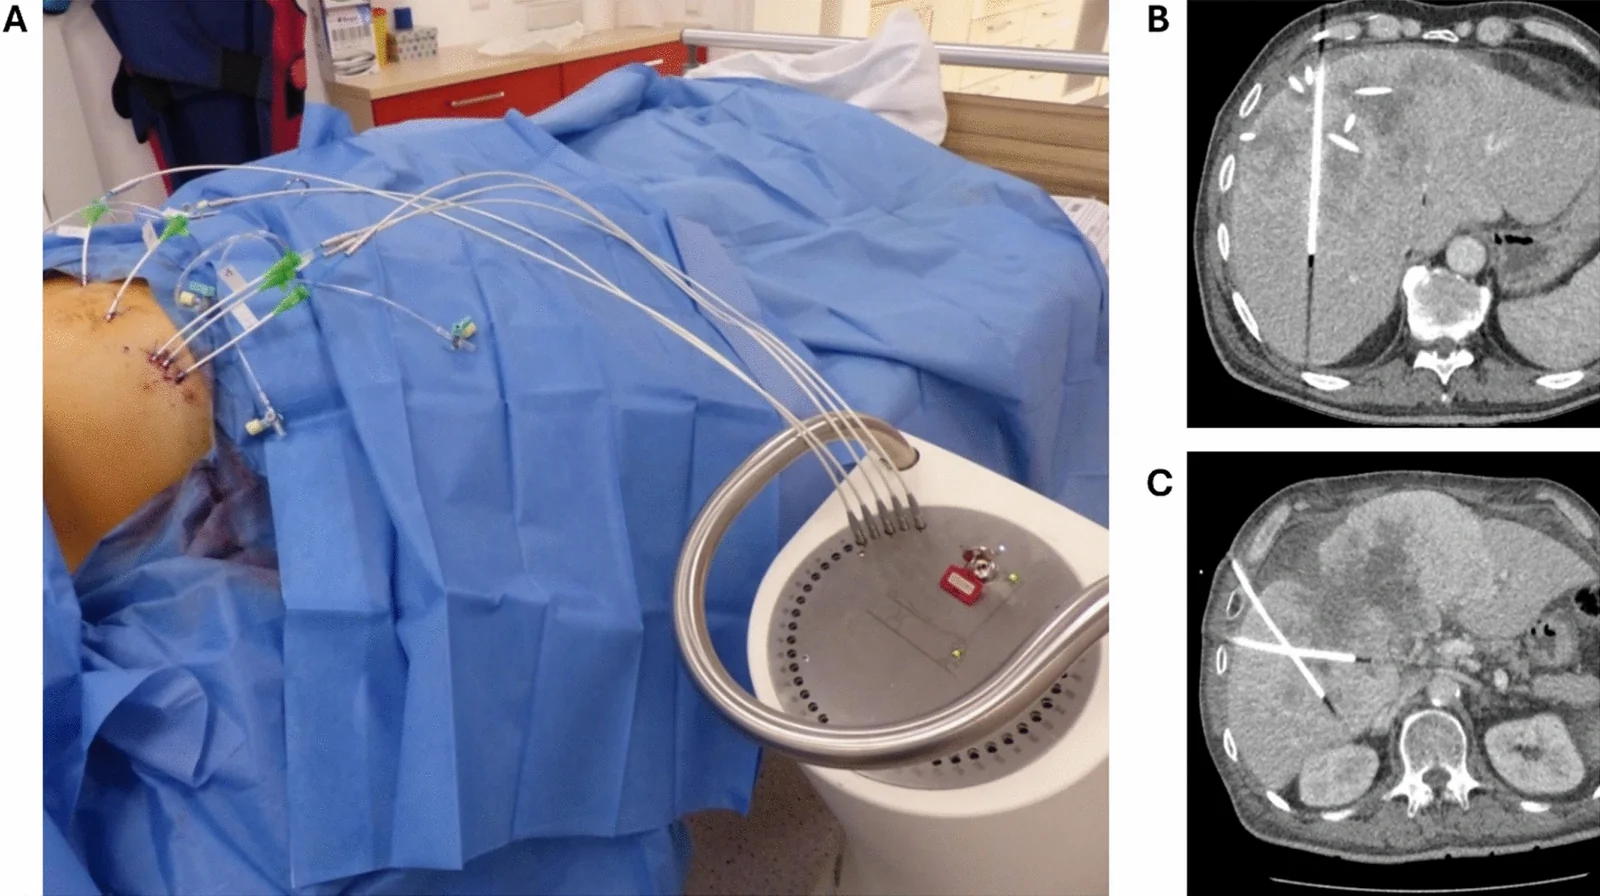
Representative images of patients undergoing HDR-BT with multiple catheters. A. patient undergoing irradiation with the applicator connected to 5 BT catheters which were placed in liver lesions. B, C. Representative CT images showing multiple BT catheters in large malignant liver lesions
